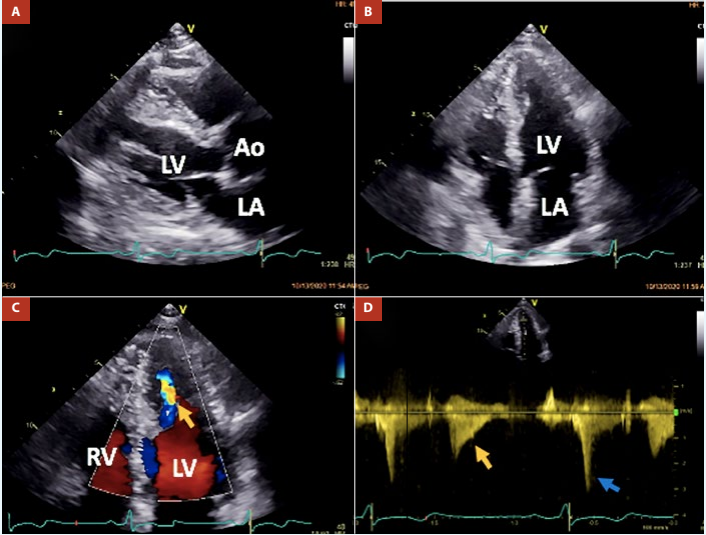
Main findings at transthoracic echocardiography in the patient. Panels A and B demonstrate hypertrophy of both the ventricular septum and lateral wall at mid-ventricular and apical levels in parasternal long-axis and apical four-chamber views, respectively. Panels C and D depict a mid-ventricular paradoxical jet flow from the apical to the basal chamber in diastole (indicated by orange arrows) and dynamic obstruction caused by head-on septum to lateral wall motion and high intra-apical end-systolic pressure (indicated by the blue arrow).
